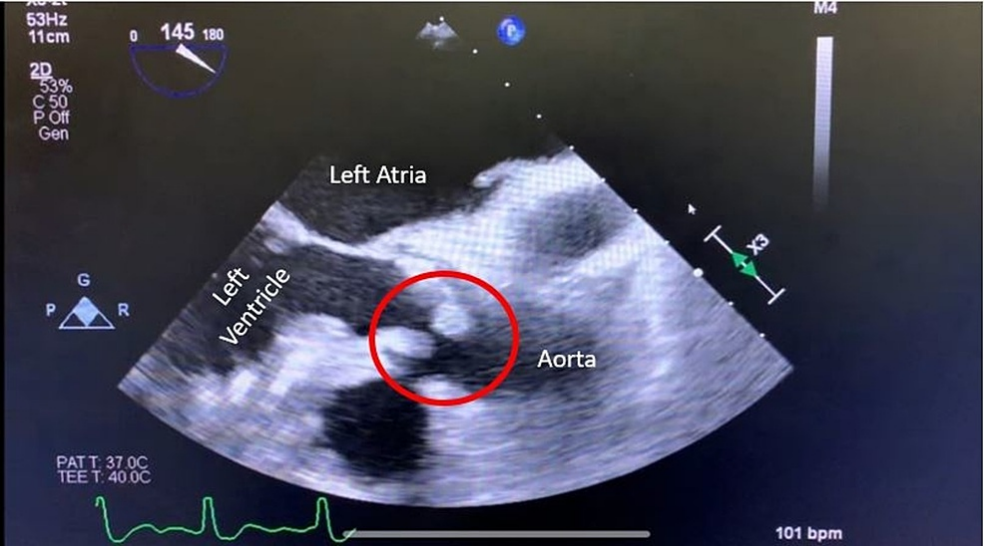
Transesophageal echocardiogram three-chamber view with aortic valve vegetation circumscribed
